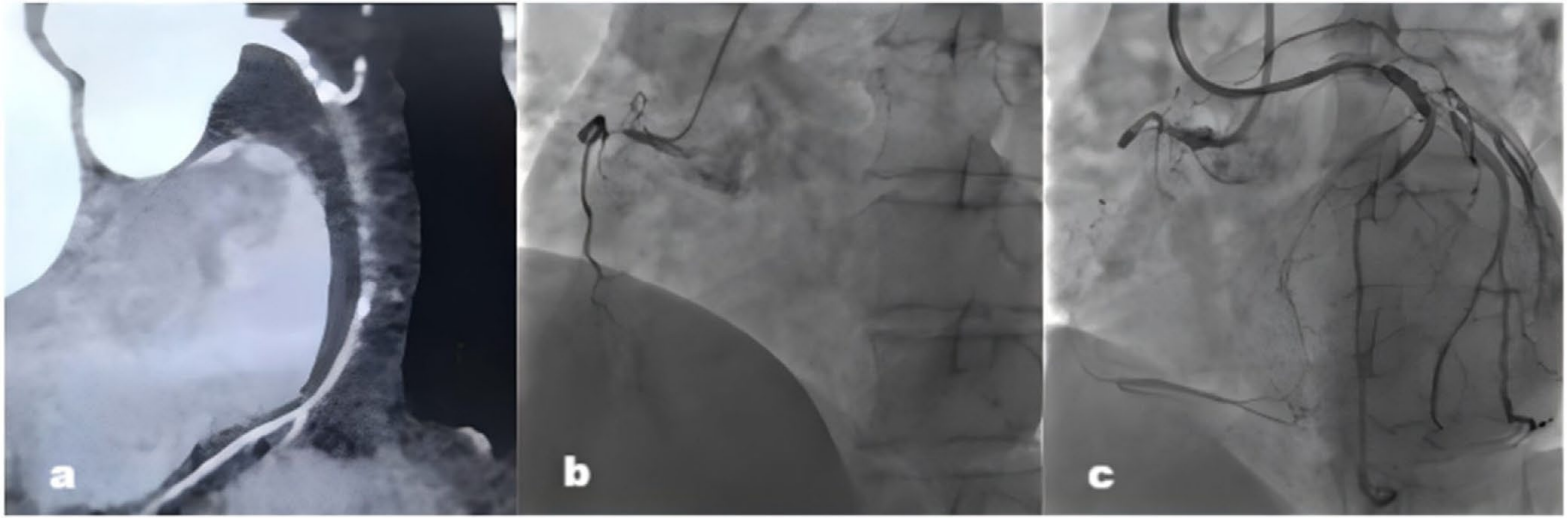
Coronary computed tomography angiography revealed calcification at the entry of occlusion and the length of the occlusion > 20 mm (a). Coronary angiography showed complete occlusion of the right coronary artery with a blunt entry, bending > 45° (b). The left anterior descending artery and left circumflex artery supplied tortuous and poorly developed collateral branches to the right coronary artery (c).
